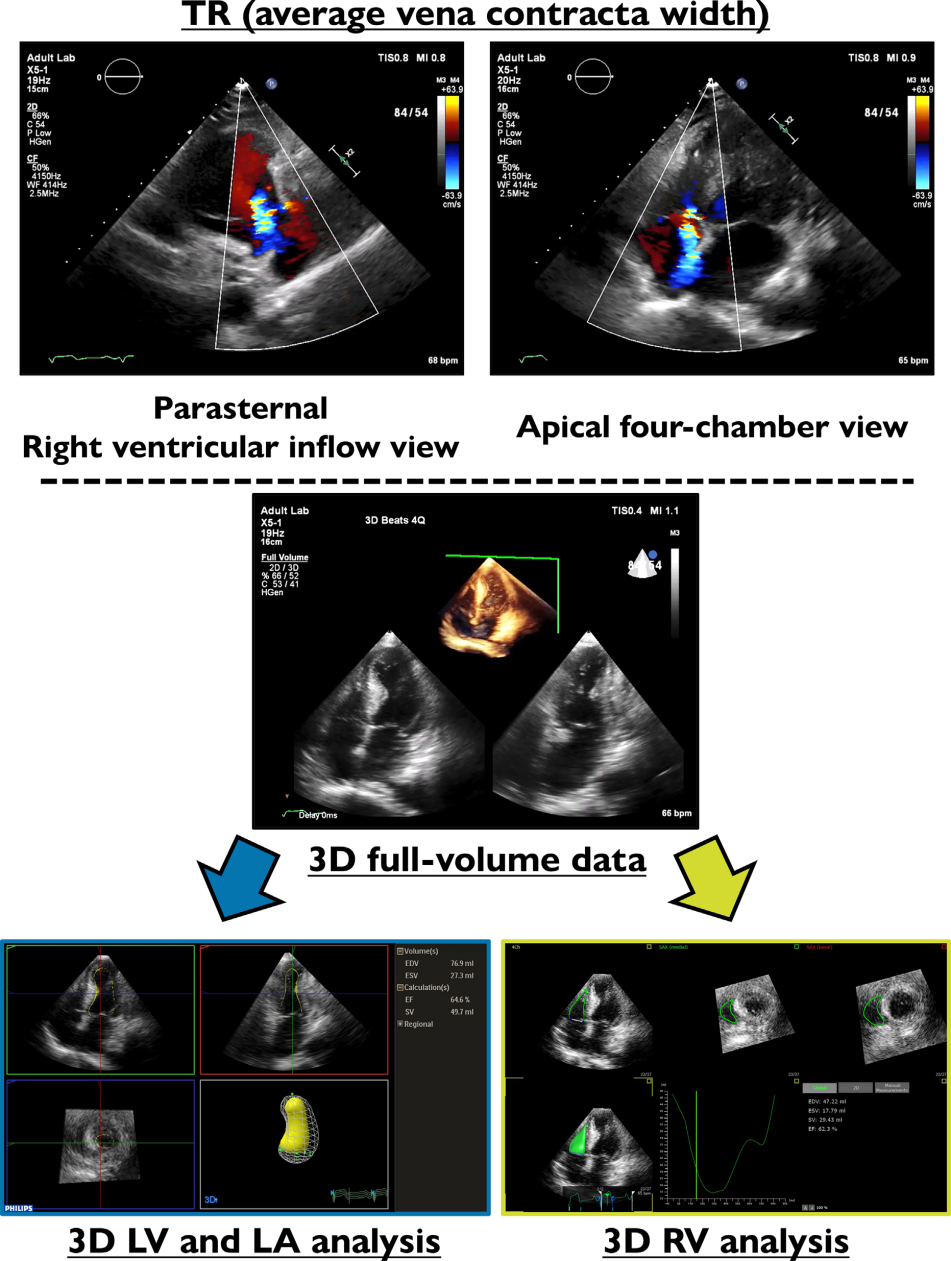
The severity of tricuspid regurgitation was semi‐quantified by the average vena contracta width on parasternal right ventricular inflow and apical four‐chamber views with colour Doppler images. Three‐dimensional full‐volume data were transferred to personal computers for offline analysis. QLAB15 3D Quantification Advanced software was used for left ventricular and left atrial volume analysis. 4D RV‐Function 2.0 software was used for right ventricular volume analysis. LA, left atrium; LV, left ventricle; RV, right ventricle; TR, tricuspid regurgitation; 3D, three‐dimensional.
